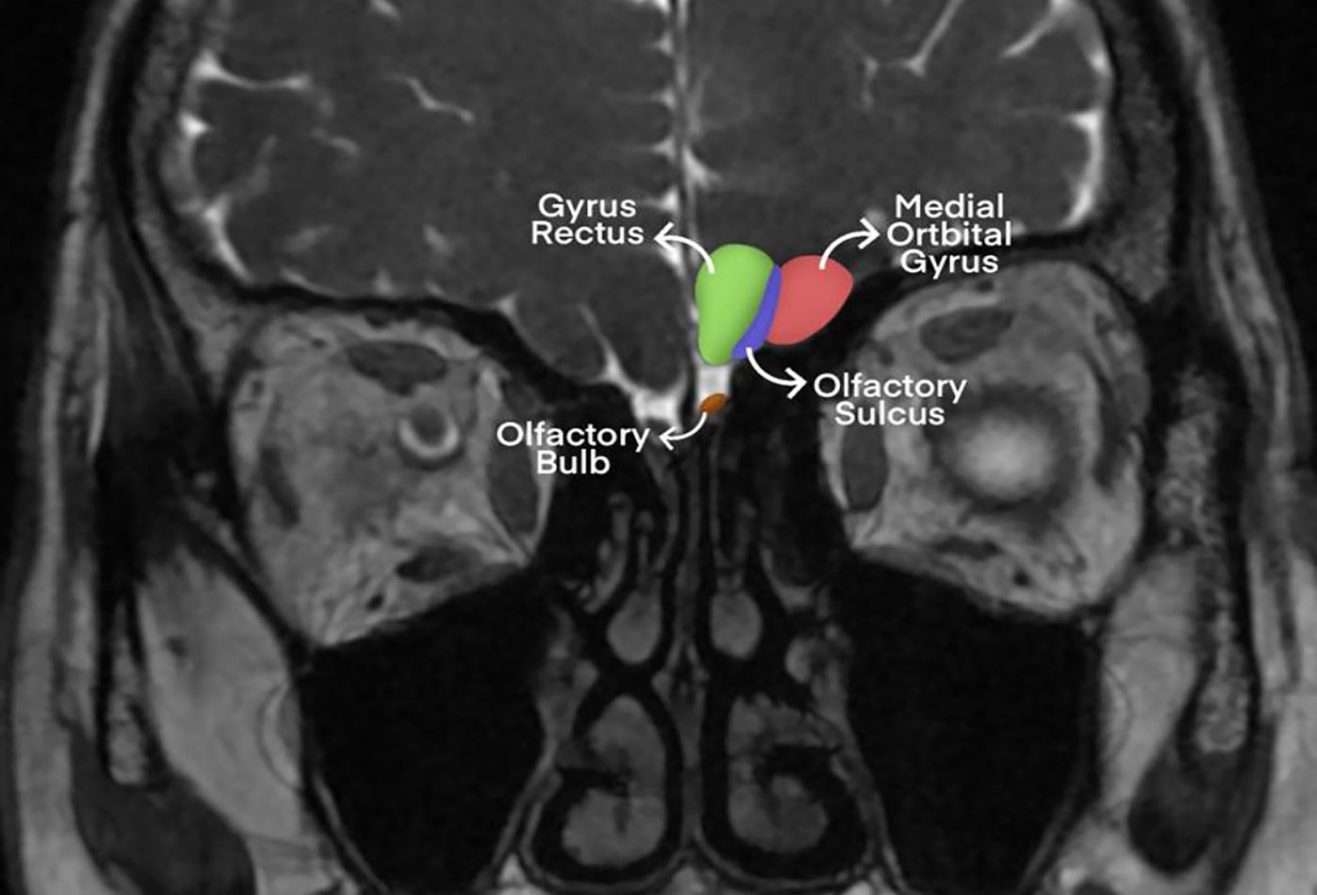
Olfactory system structures. Coronal plane MR image of the olfactory system: medial orbital gyrus (red), gyrus rectus (green), olfactory sulcus (blue), and olfactory bulb (brown).
